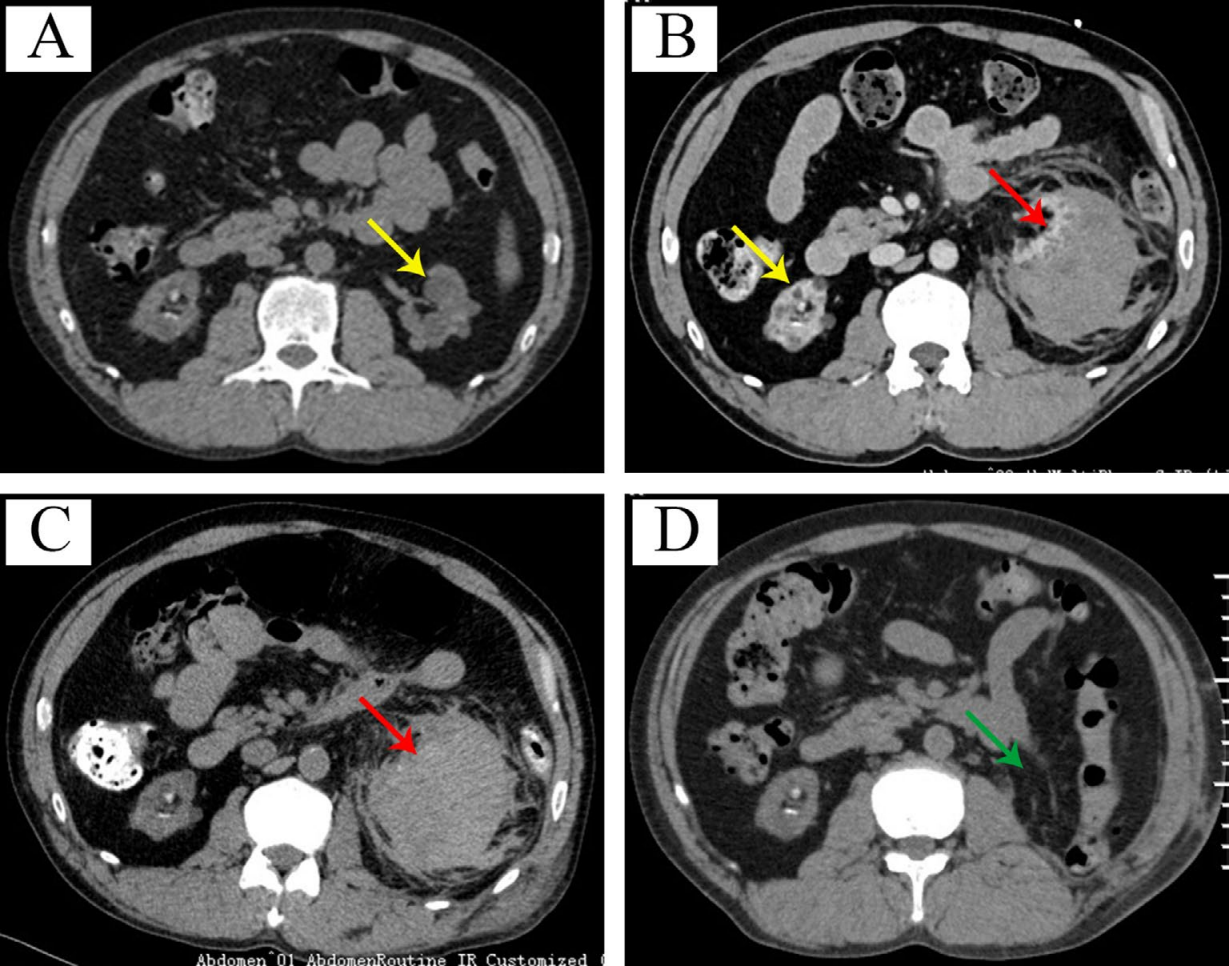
Series of CT examinations in a hemodialysis patient with spontaneous perirenal hemorrhage mimicking renal rupture. (A) CT scan dated April 7, 2024, shows bilateral kidney atrophy and multiple renal cysts (yellow arrow). A small area of hyperdensity surrounding the atrophic left kidney was considered more likely to represent an imaging artifact rather than true hemorrhage. (B) Enhanced CT performed on November 29, 2024, reveals a lobulated mixed‐density lesion in the left renal area, suggestive of a subcapsular hematoma due to suspected renal rupture (red arrow). (C) CT scan from December 2, 2024, presents a lobulated lesion with heterogeneous high and low density in the left renal area, indicative of renal rupture accompanied by hematoma formation, with increased perirenal fluid compared to the CT on November 29, 2024. (D) CT scan from January 8, 2025, shows the status post‐left nephrectomy (green arrow indicates the previous location of the left kidney).
